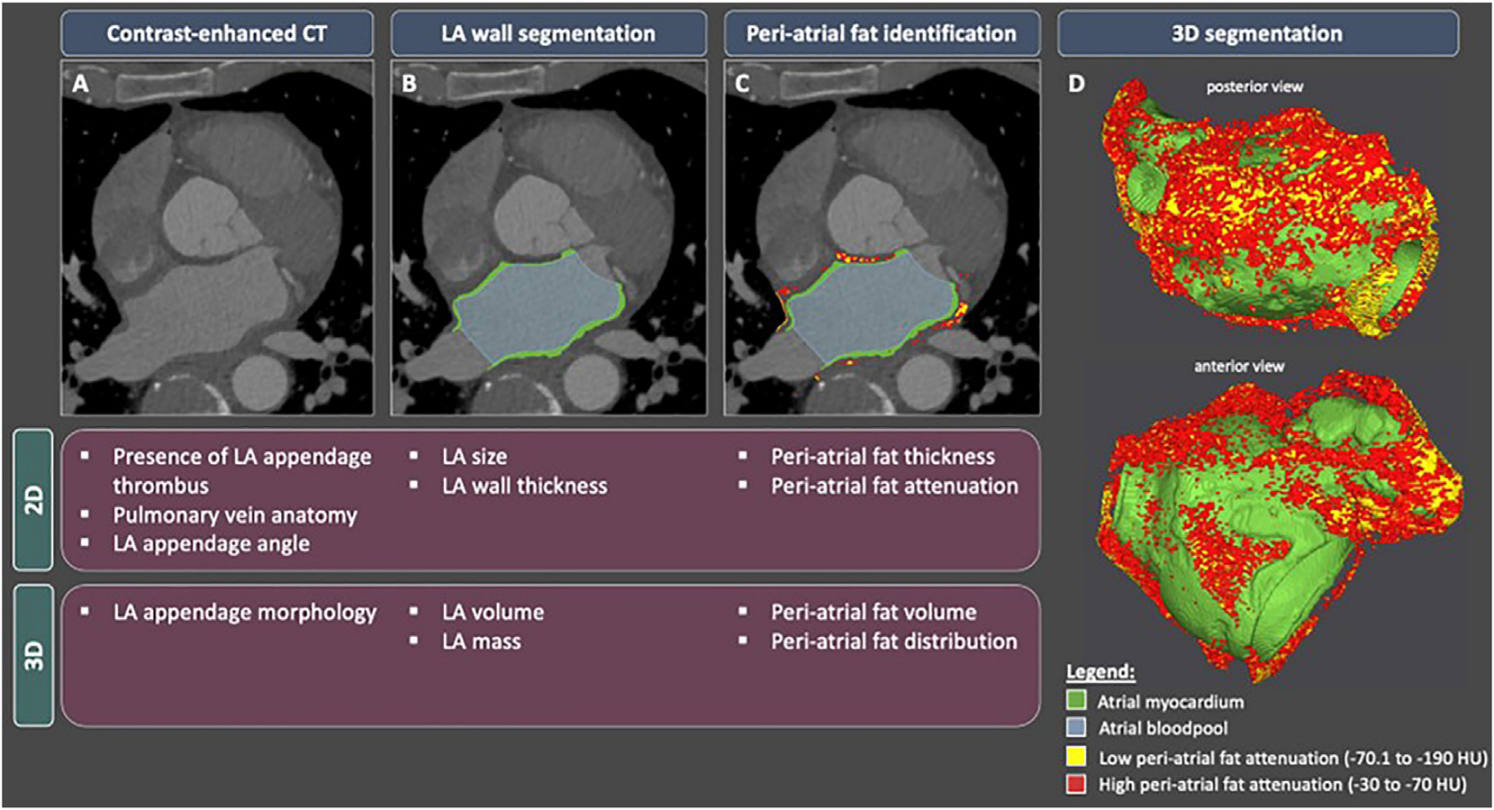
The current and potential value of computed tomography imaging in atrial fibrillation. (A) Contrast-enhanced computed tomography of the left atrium. (B) Semi-automatic segmentation of the left atrial wall and blood pool. (C) Identification of epicardial fat surrounding the left atrium where epicardial adipose tissue is defined as a hypodense layer with a density between −190 and −30 Hounsfield units. (D) Volumetric assessment of left atrium, peri-atrial fat and geographical distribution of peri-atrial fat and associated attenuation. CT, computed tomography; FAI, fat attenuation index; LA, left atrium; HU, Houndsfield units.
